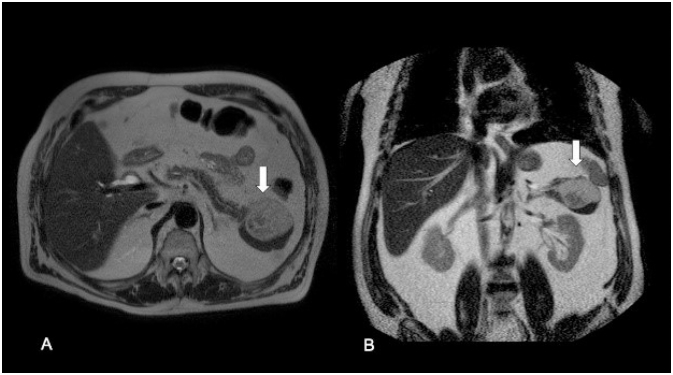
T2-weighted images. (A) Axial. (B) Coronal. Heterogeneous and well-defined solid mass in pancreatic tail (white arrows). No ductal.
